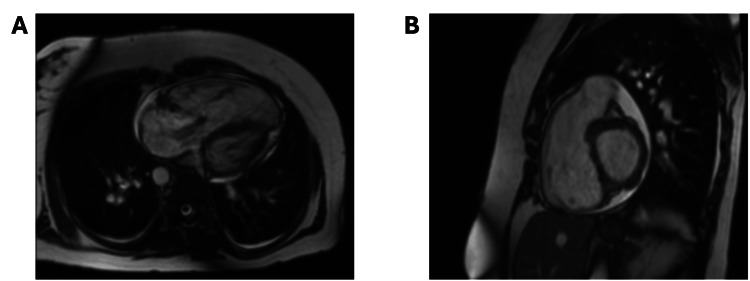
Cardiac MRI demonstrating severe RV dilation. (A) Four-chamber view showing marked enlargement of the RV relative to the LV. (B) Short-axis view demonstrating RV dilation with associated RV free-wall thickening, consistent with chronic pressure overload. LV, left ventricle/left ventricular; RV, right ventricle/right ventricular
